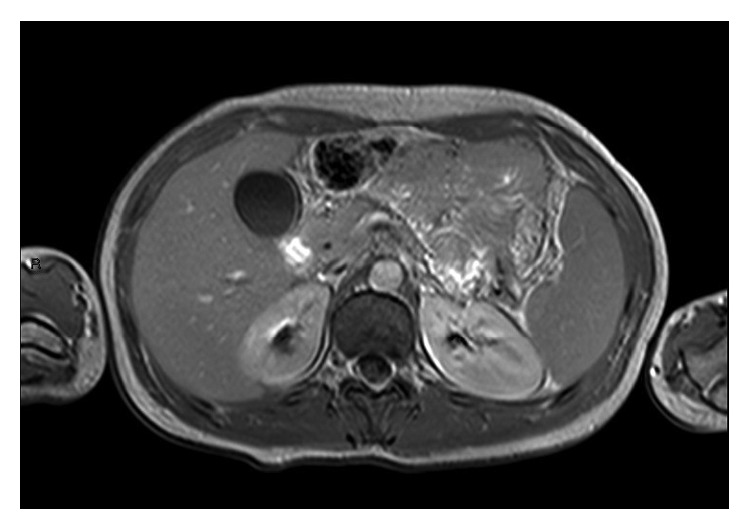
MRI showing normal spleen.
